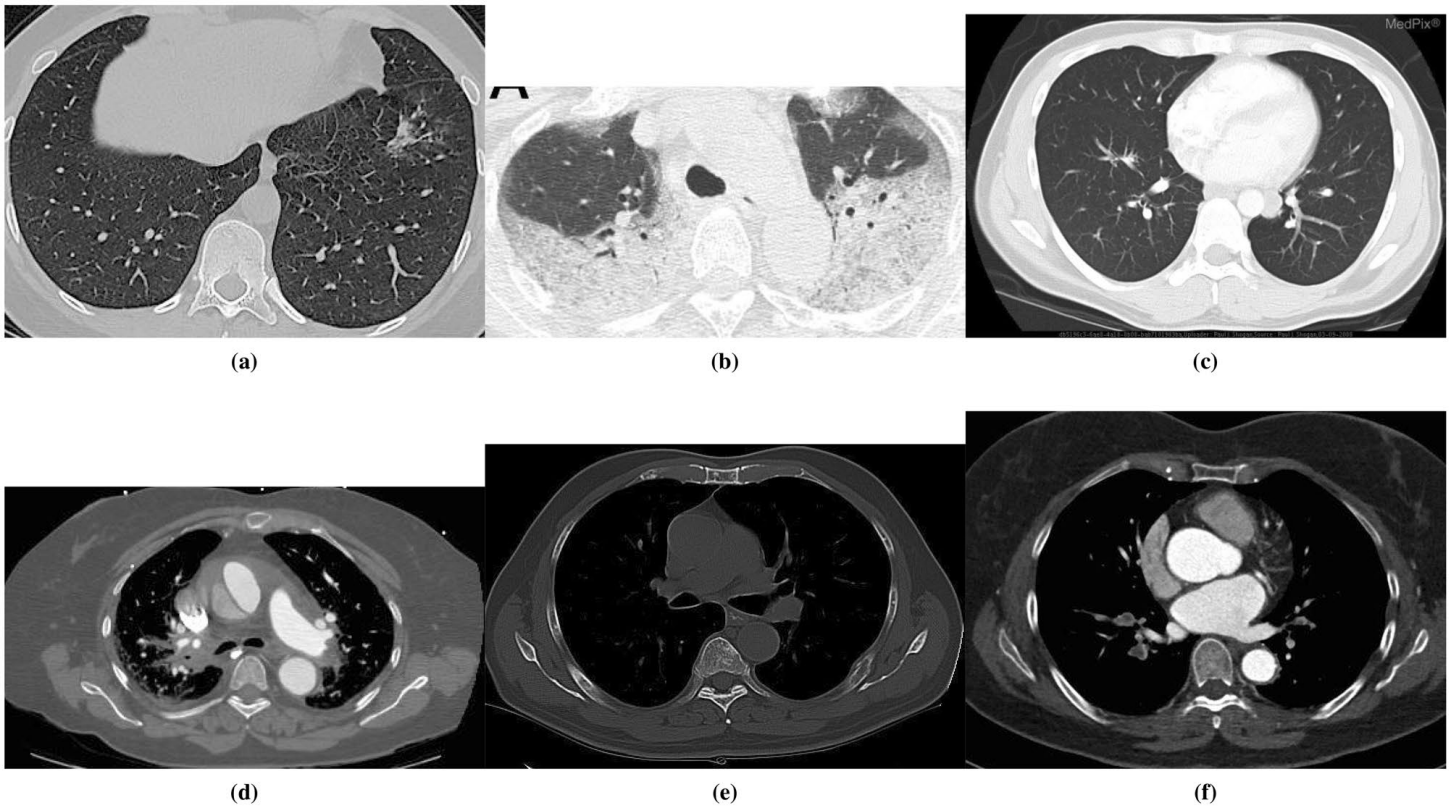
Several instances of COVID-CT dataset: The images come with diverse illumination, format, and shapes.
